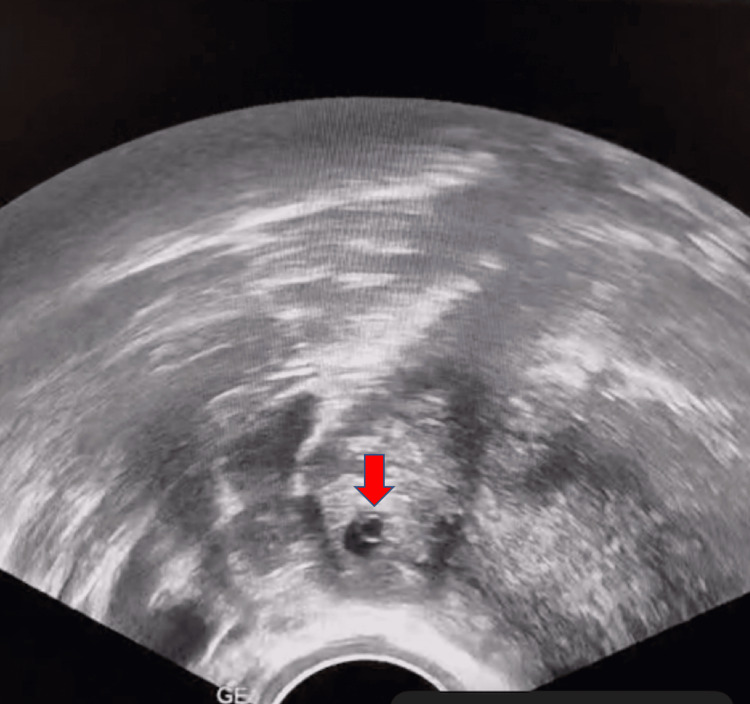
Transvaginal ultrasound revealed a gestational sac embedded in the cesarean scar (red arrow).
